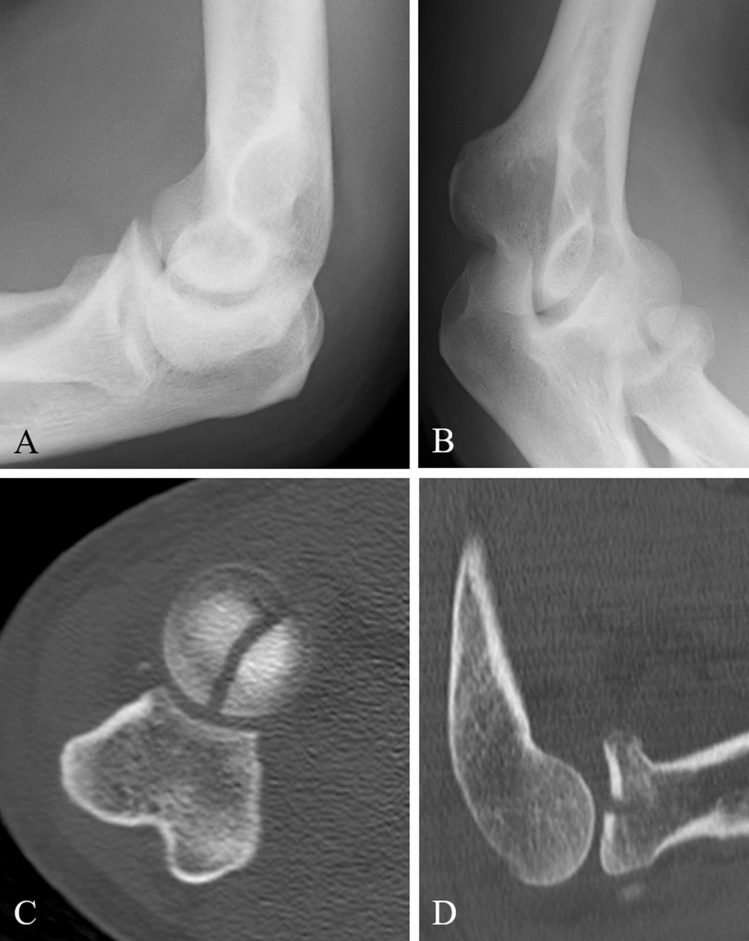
a, b Preoperative radiographs (lateral and oblique) of a Mason type II fracture (Case 3). c CT-scan in axial view and d in sagittal view
